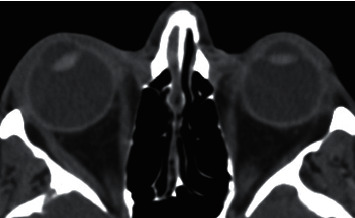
Open globe injury in a 24-year-old man. Axial unenhanced CT image showing anterior chamber deepening and dislocated lens in the right eye.
